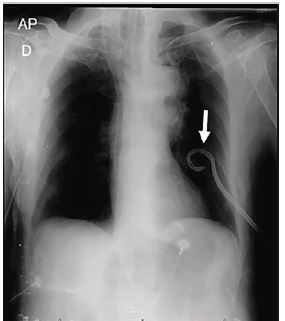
Chest radiograph: well-positioned catheter and good lung expansion (arrow).
